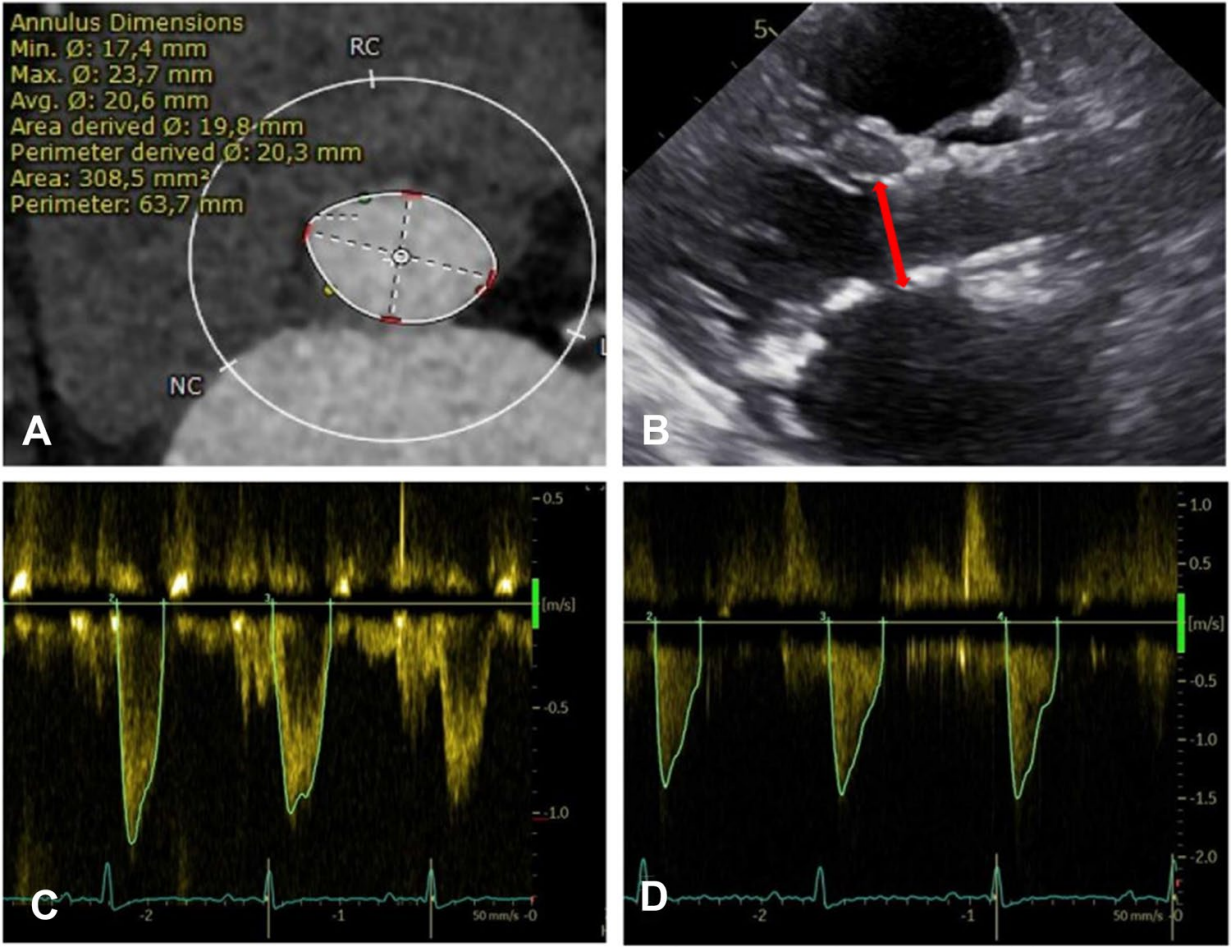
Multisliced Computed Tomography and Echocardiographic Measurements. A The Aortic Annulus was measured at the nadirs of the coronary cusps. B The left ventricular outflow tract (LVOT) (red line), used for the calculation of effective orifice area, was measured from the outer-to-outer border of the ventricular side of the stented valve. C Continuous wave doppler was measured across the aortic valve. D A pulsed-wave Doppler sample was placed in the LVOT to measure the LVOT velocity time integral
